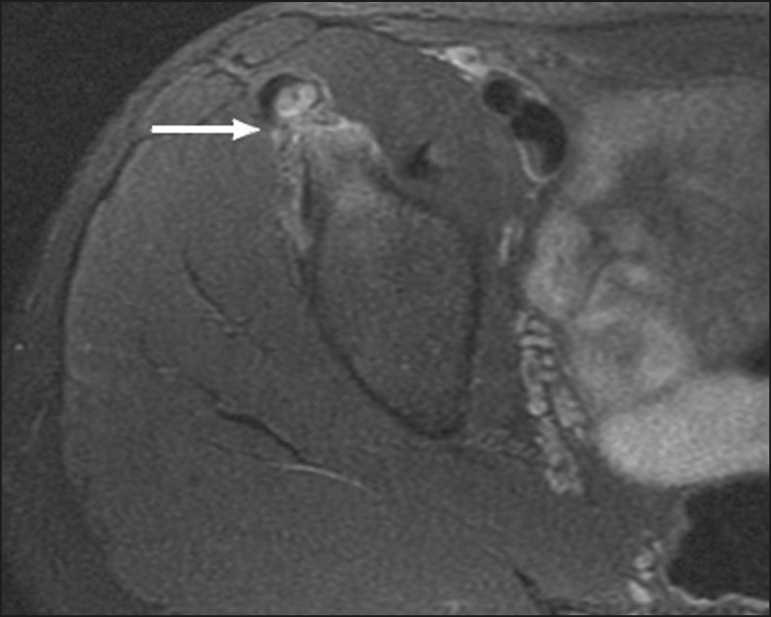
A 14-year-old male. Axial proton density-weighted fat-saturated MRI showing bone edema in the right anteroinferior iliac spine.
